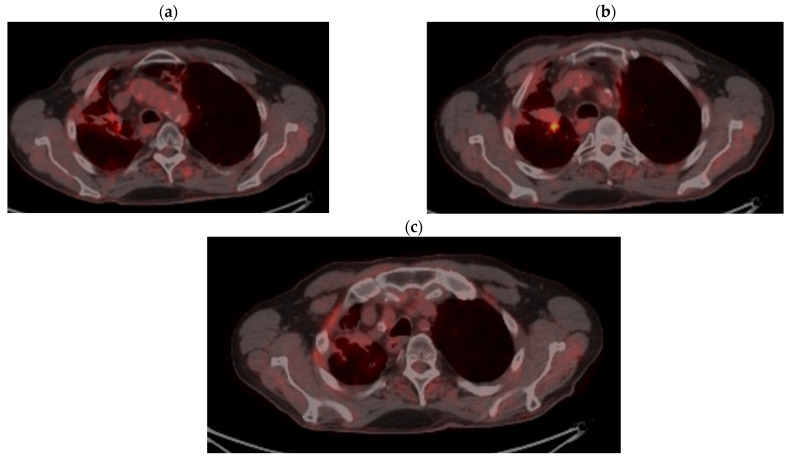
(a–c) Reevaluation of PET CT from 11/17/2021 vs. PET CT from 03/2018.
